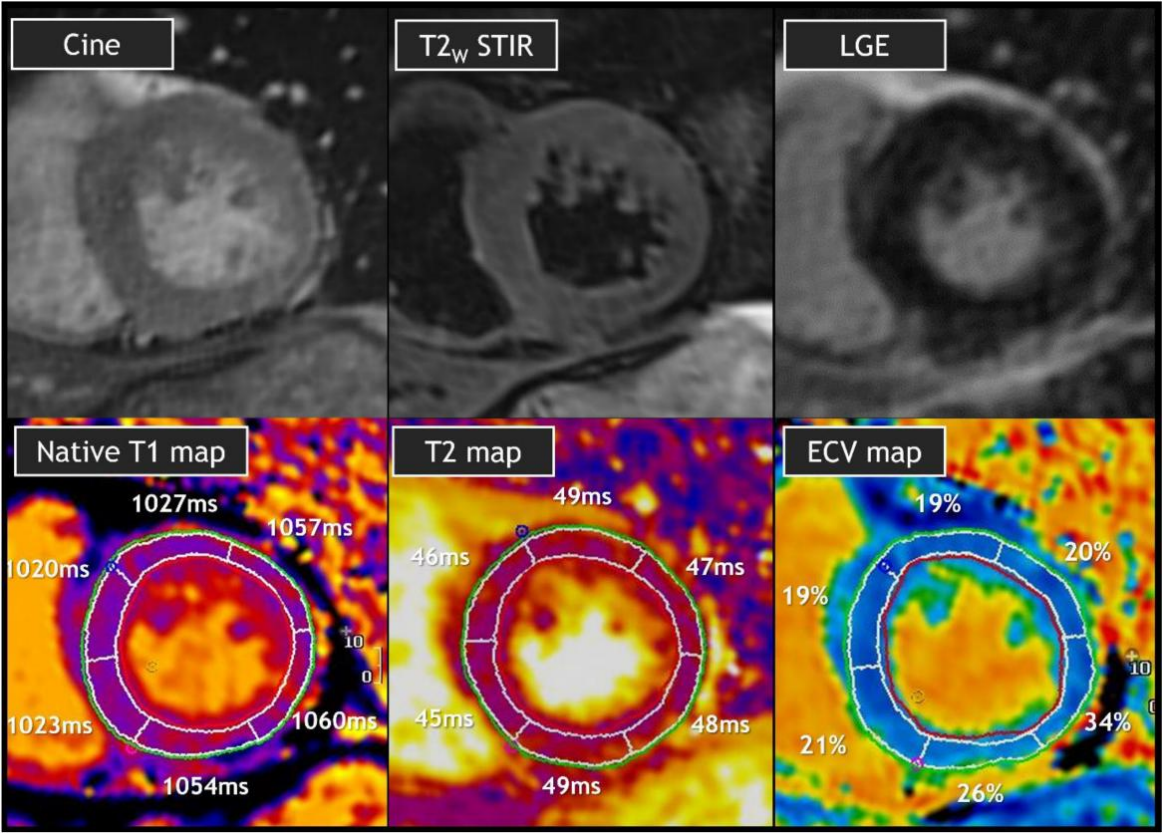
MRI findings. Exemplary MRI images showing normal to slightly increased left ventricular wall thickness on cine image, no clear evidence of focal oedema on T2w STIR image, and no clear focal fibrosis on LGE image. Furthermore, quantitative parametric MRI images neither showed clear evidence of global fibrosis with normal to borderline elevated T1 values (mean 1026 ms, normal <1052 ms) and ECV values (mean 22%, normal 20%–29%), nor evidence of global oedema with normal to borderline elevated T2 values (mean 47 ms, normal values <50.3 ms). ECV, extracellular volume; LGE, late gadolinium enhancement; ICI, immune checkpoint inhibitor, late gadolinium enhancement; T2w STIR, T2-weighted short tau inversion recovery
